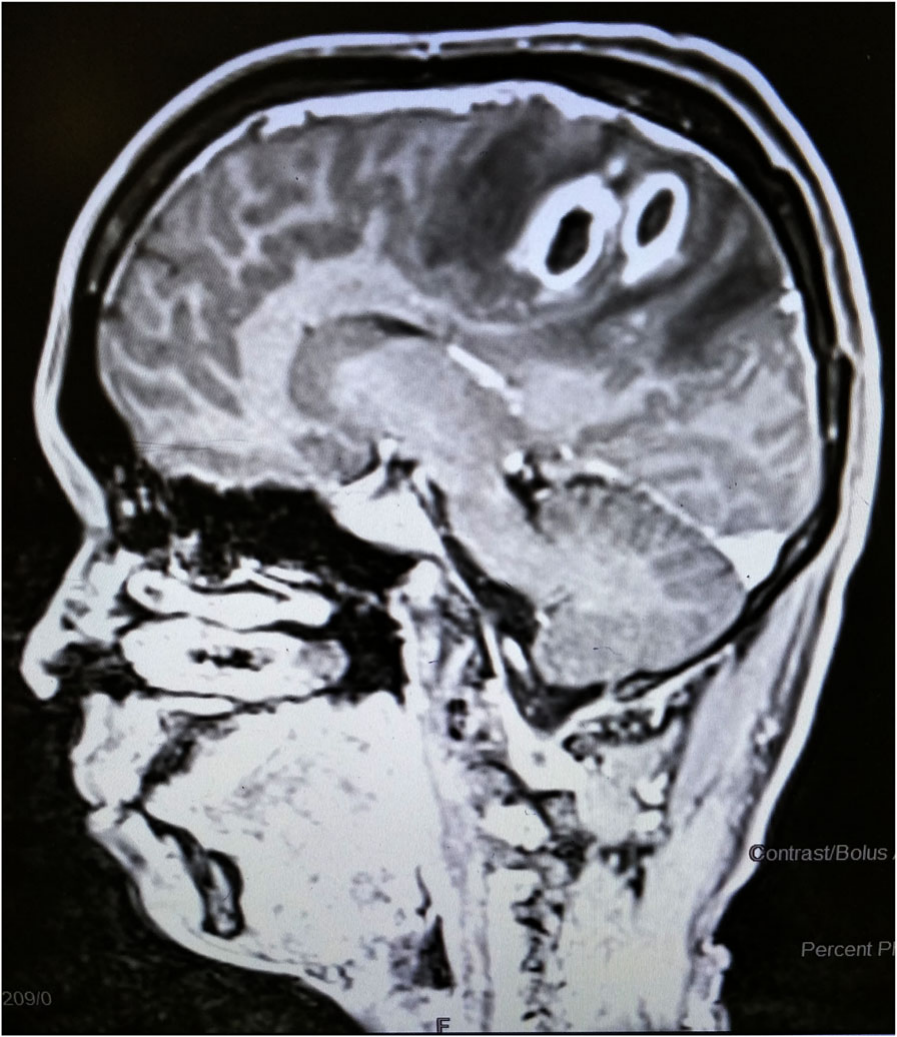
MRI of the brain showing a bi-lobed rim enhancing lesion due to conglomeration of two adjoining rounded lesion in the right parietal parasagittal region
